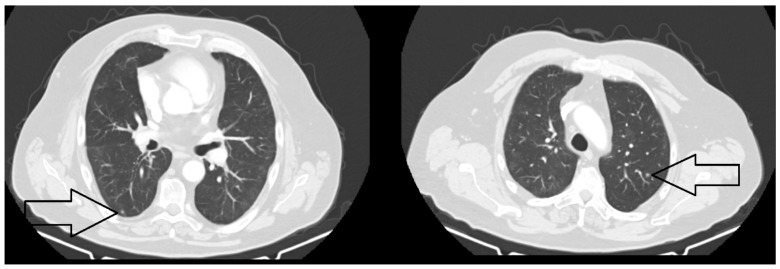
The CT scan of the thorax with contrast medium—October 2021. A few newly occurring micronodules and small pulmonary nodules (black arrows) appeared in the right Fowler segment, respectively, of the upper lingula (9/7 mm).
